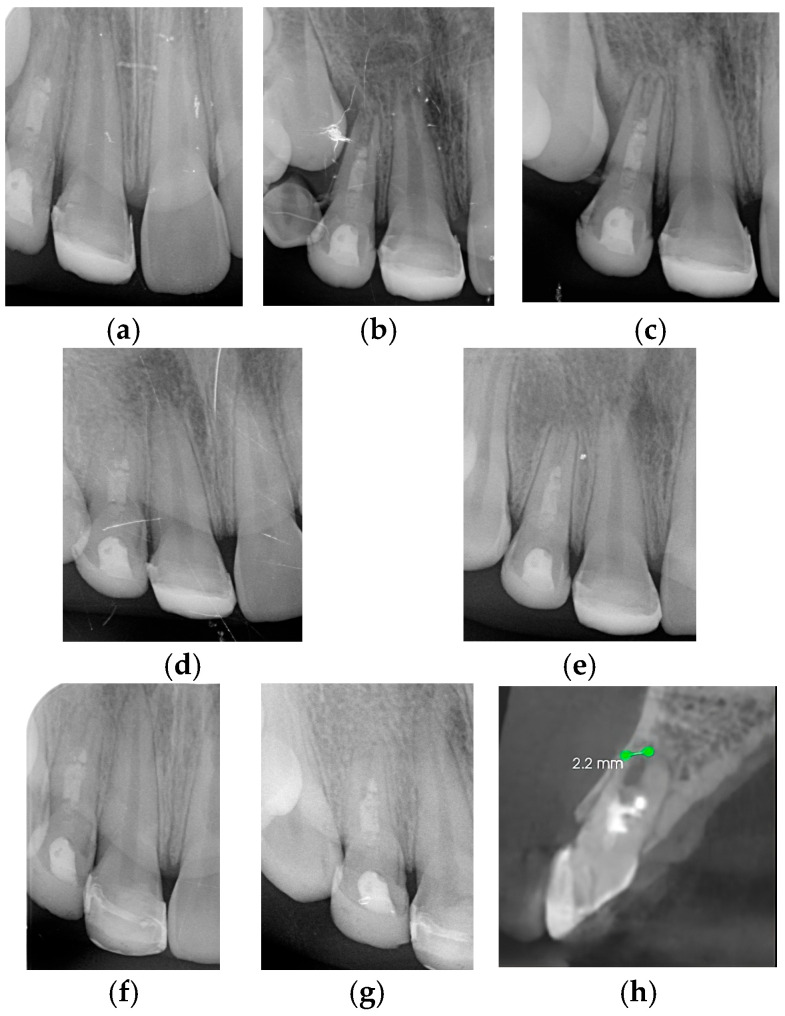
Clinical Case 1. (a) Periapical radiograph taken at 6-month follow-up. (b) Periapical radiograph taken at 1-year follow-up. (c) Periapical radiograph taken at 2-year follow-up. (d) Periapical radiograph taken at 3-year follow-up. (e) Periapical radiograph taken at 4-year follow-up. (f) Periapical radiograph taken at 5-year follow-up. (g) Periapical radiograph taken at 6-year follow-up. (h) CBCT taken at 6-year follow-up.
